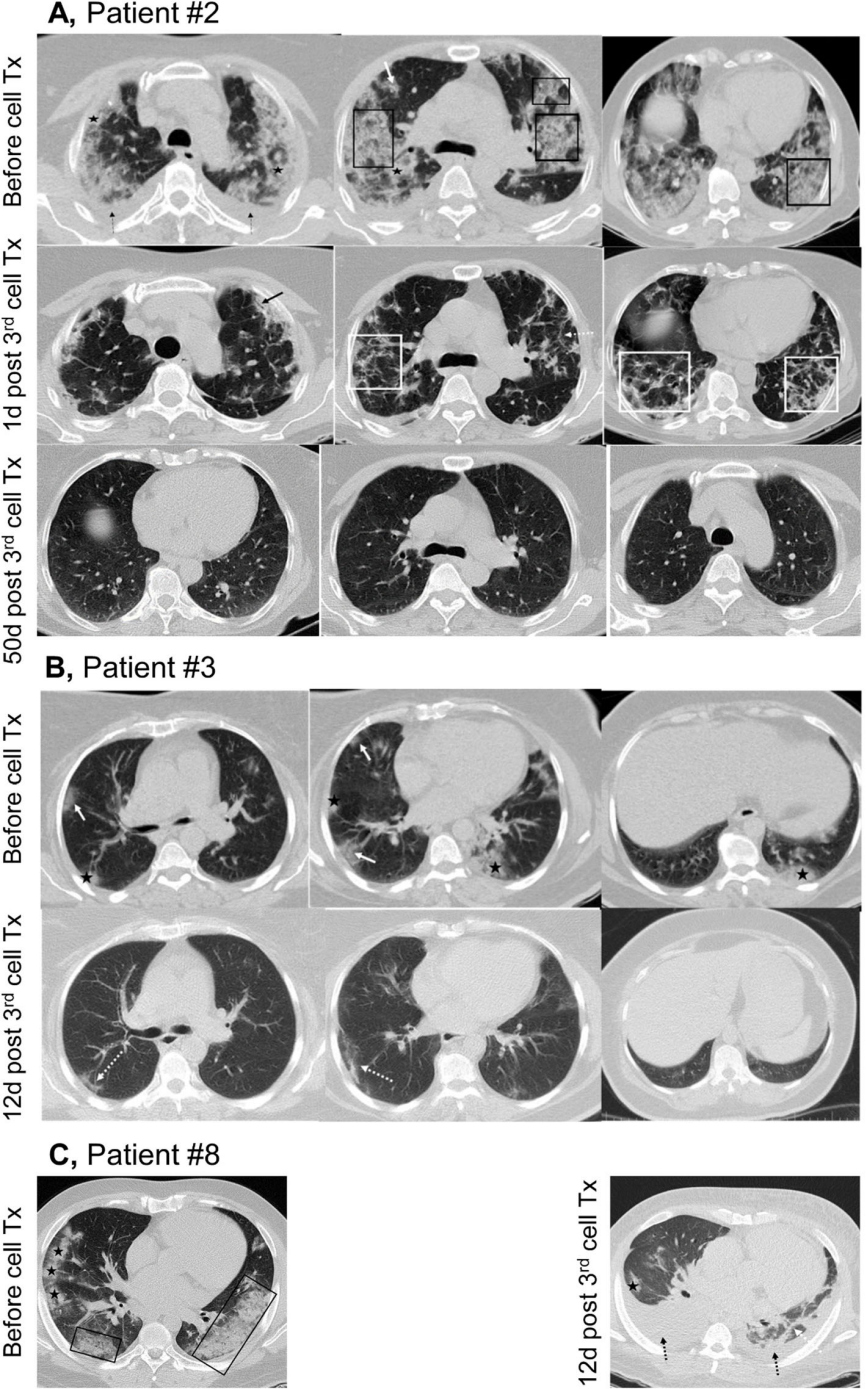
Chest computed tomography (CT) images in three survivors. a Patient #2: First row is prior to cell infusion. Note the extensive mixed ground glass opacities, crazy paving appearance, vascular dilatation, consolidations with peripheral subpleural dominancy, and bilateral mild pleural effusion. The CT severity score for all five lobes was 24. The second row shows CT images 1 day after the third dose of cell therapy. There is a decrease in extension of consolidations associated with band-like opacities and traction bronchiectasis are evident. CT severity score for all five lobes was 18. The third row shows near complete resolution of opacities and subpleural bands without residual fibrosis 50 days after MSC therapy. The CT severity score for all five lobes was 2. The percentage of lung involvement in each image of the first, second, and third columns was assessed at pretreatment, 1 day after the third post-cell therapy, and after 50 days of treatment, respectively, as follows. First column: about 60%, 25%, and 0%; second column: about 75%, 30%, and 0%; and third column: about 90%, 50%, and 2%. b Patient #3: First row shows chest CT images before cell therapy. Note the patchy areas of ground glass opacity and consolidations in the subpleural regions of the lungs. The CT severity score for all five lobes was 16. The second row shows a significant reduction in the extension of lung involvement 12 days after the third dose of cell therapy. Most consolidations had resolved completely with only band-like opacities and mild tiny residual ground glass opacities present. The CT severity score for all five lobes was 8. The percentage of lung involvement in single images from the first, second, and third columns was assessed at pretreatment and 12 days after the third cell therapy, respectively, as follows. First column: about 15% and 5%; second column: about 35% and 15%; and third column: about 20% and 3%. c Patient #8: Axial CT scan images from the base of the lung before cell therapy. The left image shows peripheral subpleural consolidations and ground glass opacity. At the same level, the right image shows a significant decrease in consolidations 12 days after cell therapy; however, the patient developed bilateral pleural effusion due to acute renal failure during the course of the disease. The CT severity score for all five lobes at the initial lung CT scan was 24, which decreased to 13 at 12 days after cell therapy. The percentage of lung involvement in the pretreatment image was about 60%, which decreased to 20% in the post-treatment image. In all images, different patterns of lung involvement have the following annotations: crazy paving appearance (black boxes), consolidation (black stars), pure ground glass opacity (solid white arrows), vascular dilatation (solid white arrows), traction bronchiectasis (solid black arrows), subpleural band (dashed white arrows), architectural distortion (white boxes), and pleural effusion (dashed black arrows)
